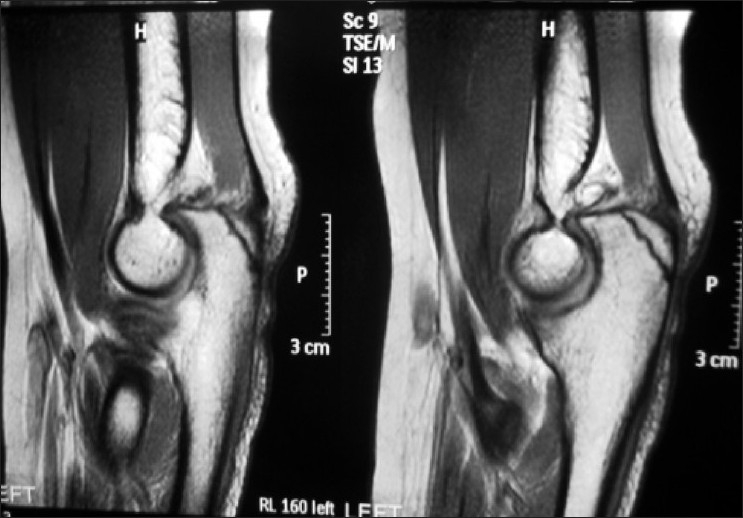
MRI scan showing presence of a loose body in the posterior compartment with a possible osteochondral defect in the dorsal articular surface of capitellum
